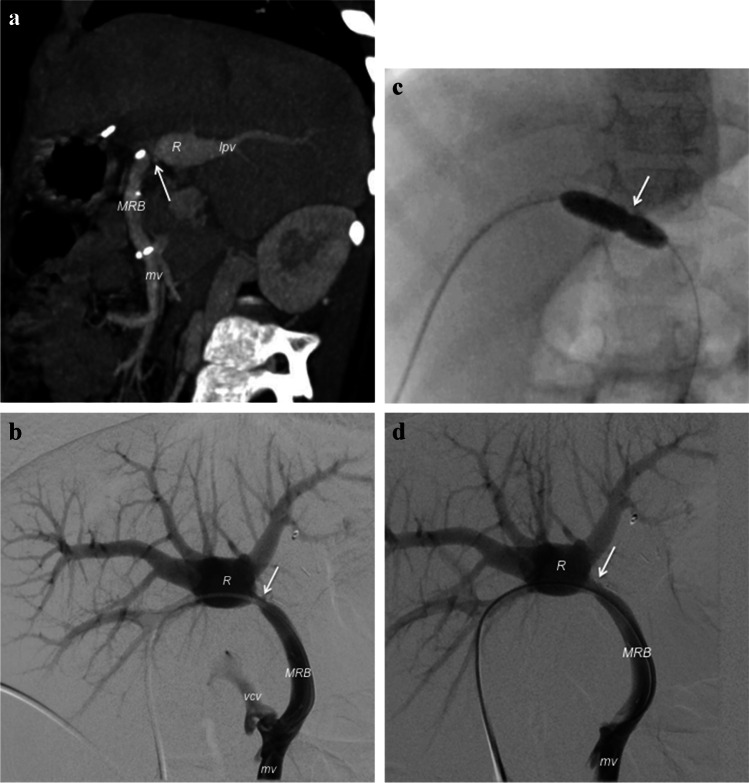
Endovascular treatment of stenotic meso-Rex bypass (MRB) in a 5-year-old girl. a Contrast-enhanced multi-detector-row sagittal CT image shows a stricture within the MRB (*arrow*). *lpv* left portal vein, *mv* mesenteric vein, *R* Rex recess. b–d Posteroanterior images during percutaneous transhepatic balloon angioplasty (digital subtraction scans in b and d). Mesenteric portogram (b) shows stenosis (*arrow*) within the left portal vein anastomosis of the MRB and vicariant collateral veins (*vcv*) arising from the mesenteric vein before the shunt. Balloon angioplasty of the anastomotic stricture (*arrow*) under fluoroscopic view (c). Fluoroscopy (d) shows the disappearance of the stenosis and collateral circulation after endovascular dilatation
